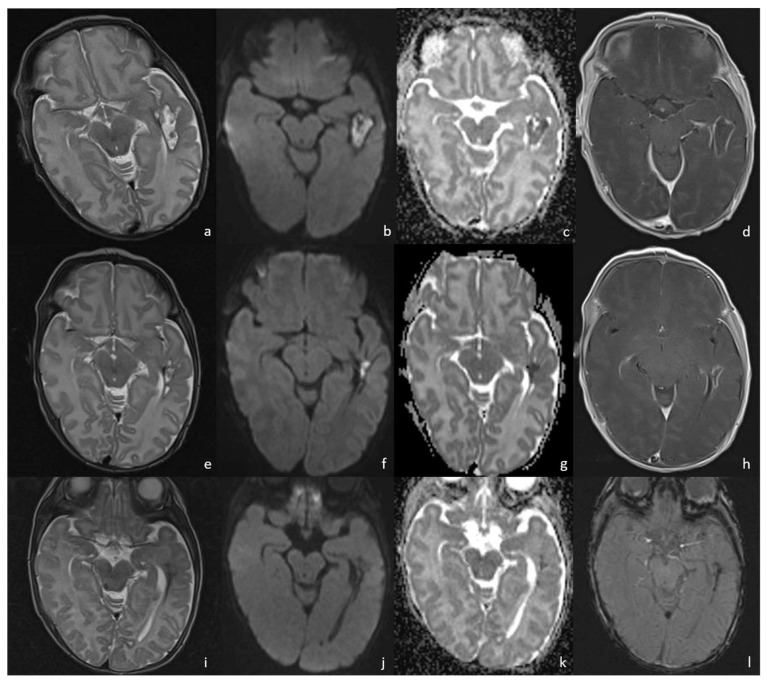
A patient affected by *Bacillus Cereus* meningoencephalitis. MRIs were acquired at 30 days, 43 days, and 63 days from birth and show the brain abscess evolution during the patient’s follow-up. At 30 days from birth (a–d), the parenchymal abscess in the left temporal lobe presents a necrotic core, appearing inhomogeneously hyperintense on T2WI (a) with diffusion restriction on DWI/ADC (b,c), and a thick capsule, appearing hypointense on T2WI with intense enhancement on post-contrast T1WI (d). At 43 days from birth, the parenchymal abscess shows a dimensional decrease and similar radiological features on T2WI (e), DWI (f), ADC (g), and post-contrast T1WI (h). At 63 days we can appreciate a hemosiderin scar appearing hypointense on T2WI (i) and SWI (l) with no diffusion restriction on DWI/ADC (j,k).
